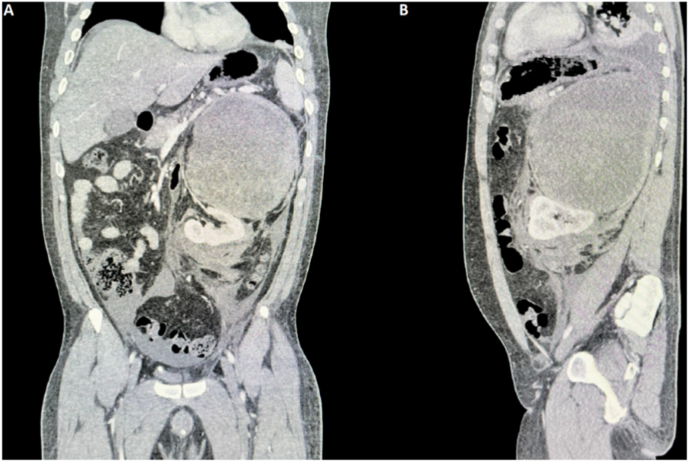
Abdominopelvic triphasic CT scan. A: Coronal view demonstrating the inferomedial displacement of the left kidney and its pedicle. B: Sagittal view.
